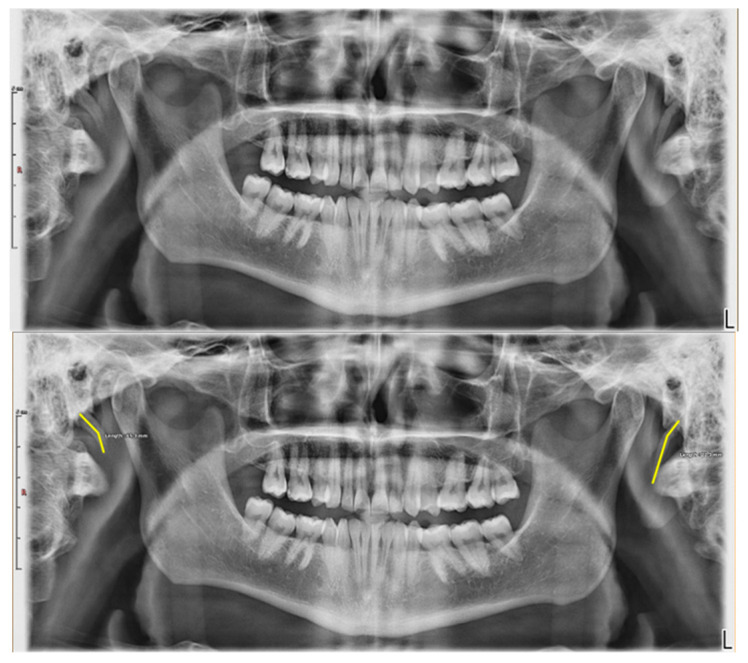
Panoramic radiographs showing styloid processes with normal length.
